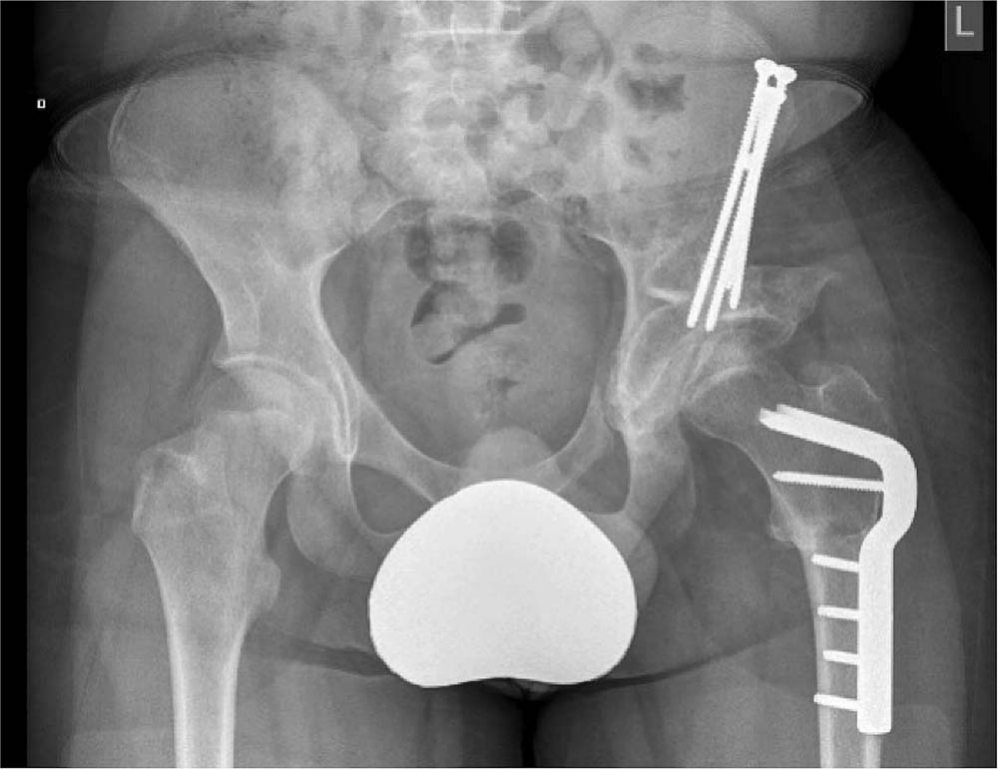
Case 1 post-operative radiograph.
